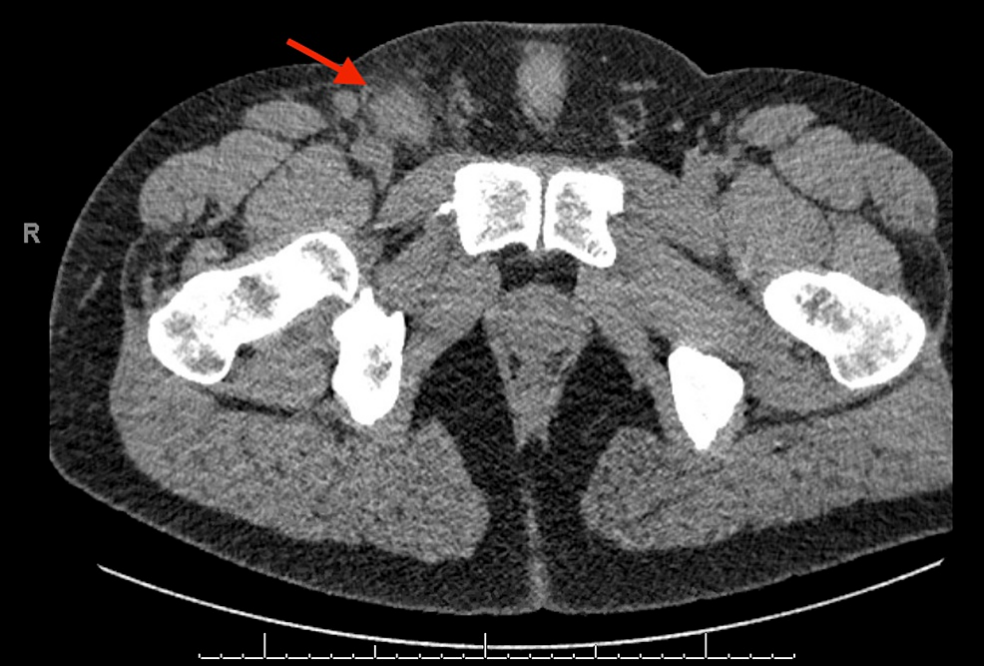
CT abdomen and pelvis (axial view) The red arrow shows enlarged right inguinal lymph nodes with surrounding inflammation concerning lymphadenitis.
